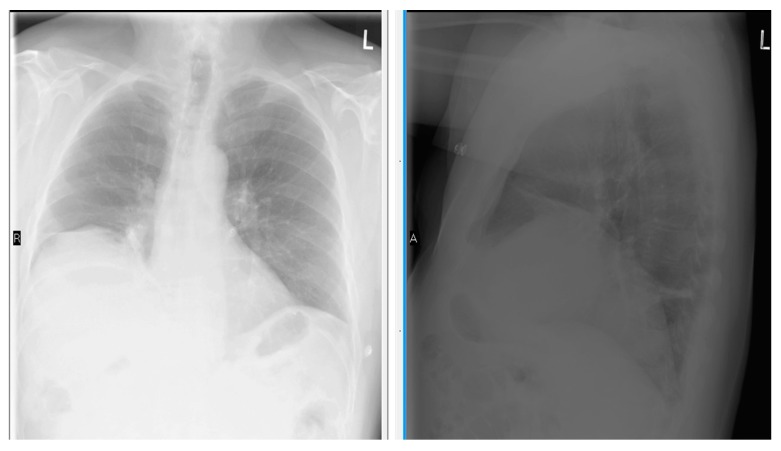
Chest X-ray Posterior-Anterior and Lateral view showing right diaphragm located more than 2 intercostal spaces compared to left side.
